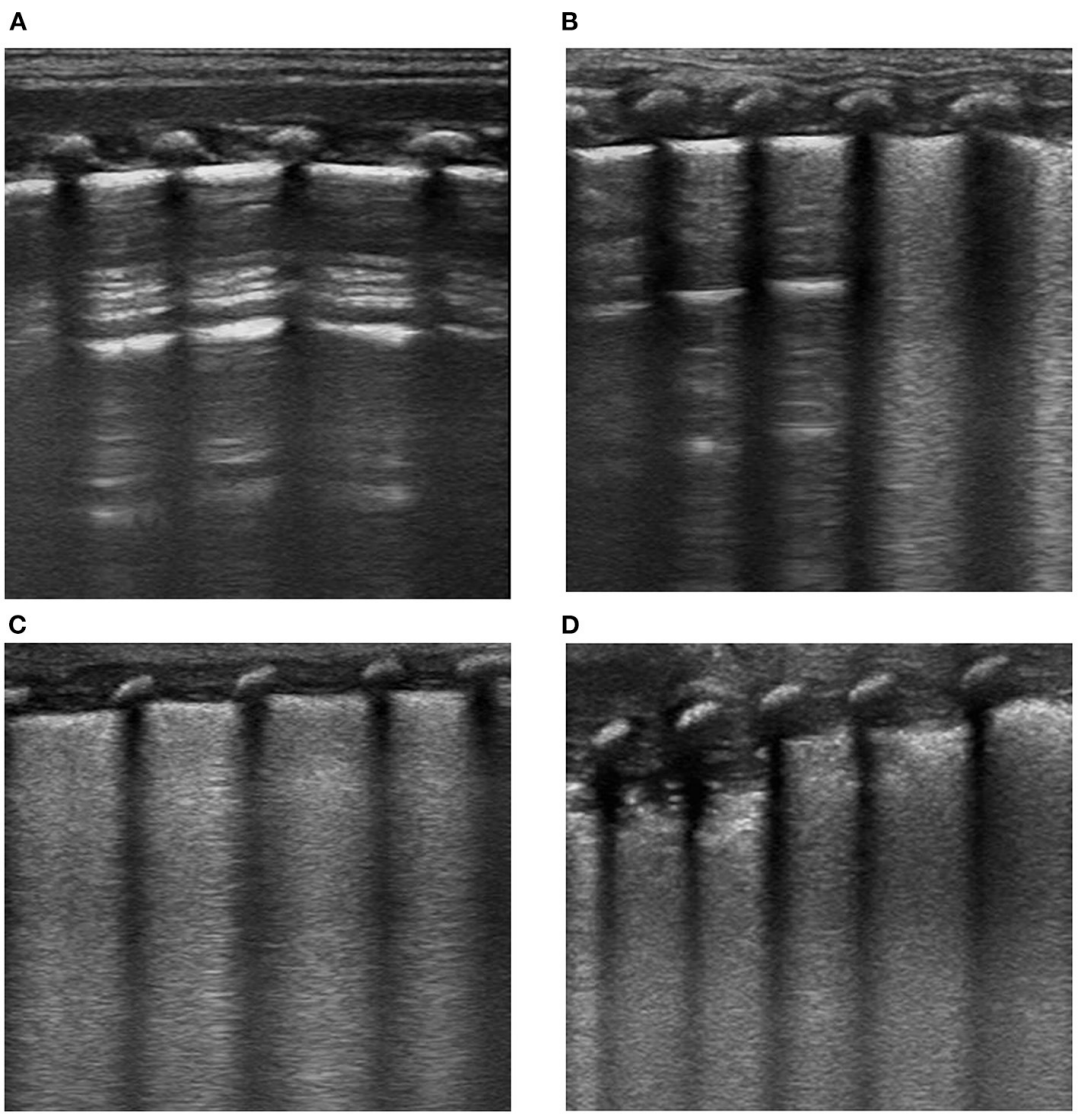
Imaging examination of lung ultrasound in newborns with RDS. (A) Score = 0, (B) score = 1, (C) score = 2, and (D) score = 3.
